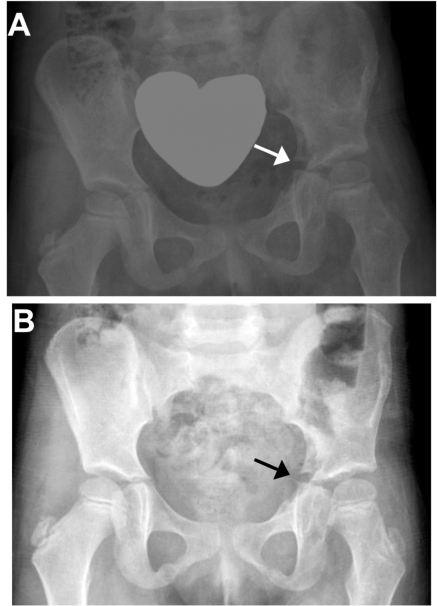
Pelvic X-ray at 4 months (A) and one year (B) after physeal bar resection shows a wide open spacing without signs of recurrent premature closure of the tri-radiate cartilage (arrows).
